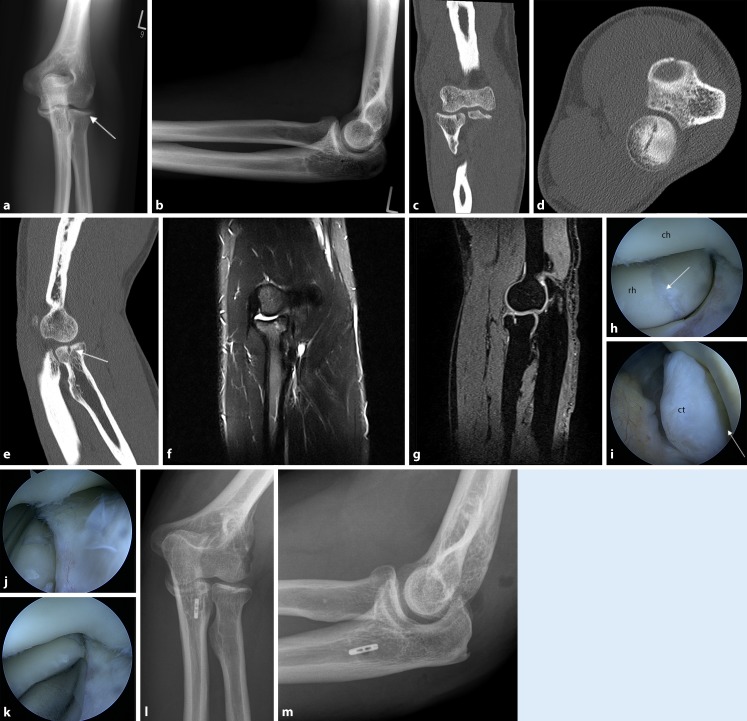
Case report: A 50-year-old patient with a radial head fracture (Mason I) after a fall had persistent symptoms after initial conservative treatment for more than 1 year. Radiography and computed tomography images show no step formation (*arrows*) at the radial head (a–e). Magnetic resonance imaging confirms the diagnosis of a Mason I fracture, but no soft tissue damage can be detected (f, g). The intraoperative results 1 year posttrauma show the former fracture (*arrow*) at the radial head (*rh*) and a gaping humeroradial joint gap (*arrow*) as an indication of lateral instability (*ch* capitulum humeri*,*
